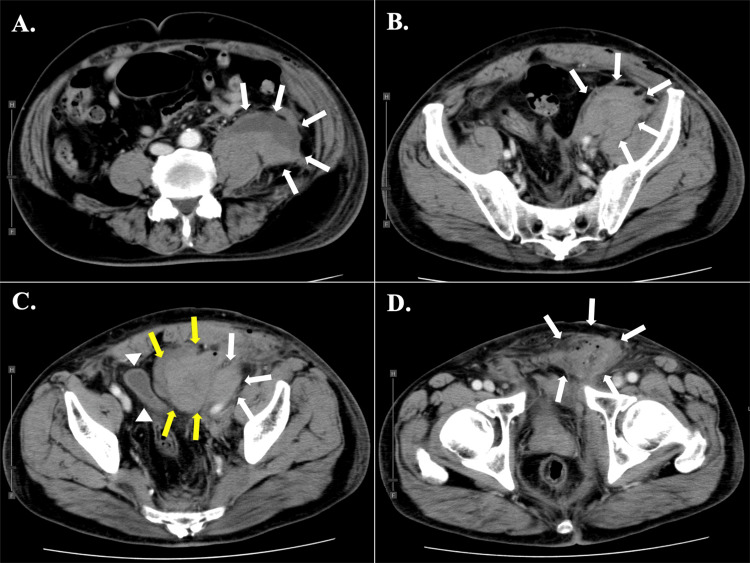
Contrast-enhanced CT images on postoperative day 2 after left inguinal hernioplasty using PHS (A, B) Massive retroperitoneal hematomas from the retropubic space to the lower retroperitoneal space were detected (white arrows). (C) The hematoma was contiguous to the preperitoneal space (yellow arrows) and severely compressed the urinary bladder (white arrowheads). (D) A contiguous left groin hematoma was also detected (white arrows). PHS: Prolene^®^ Hernia System.
